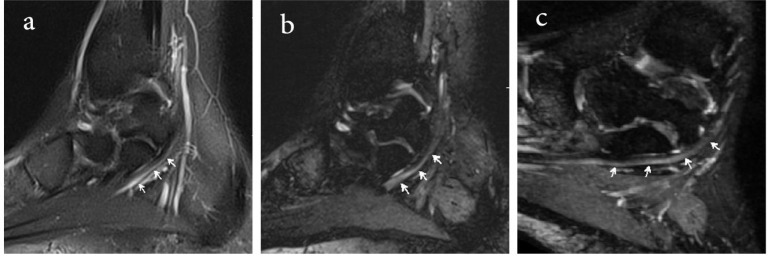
MR neurography of the distal lower extremity in a 26-year-old male The identification of the medial plantar nerve (arrows) is poor on the sagittal fat-suppressed T2-weighted image due to adjacent T2 hyperintense vessels (a), and the conspicuity score is 1. In the 3D DW-PSIF image (b), the identification of the medial plantar nerves (arrows) is definite, and the conspicuity scores is 2. In the isotropic reconstructed oblique sagittal (c) image, the medial planter nerve (arrows) can be easily depicted.
